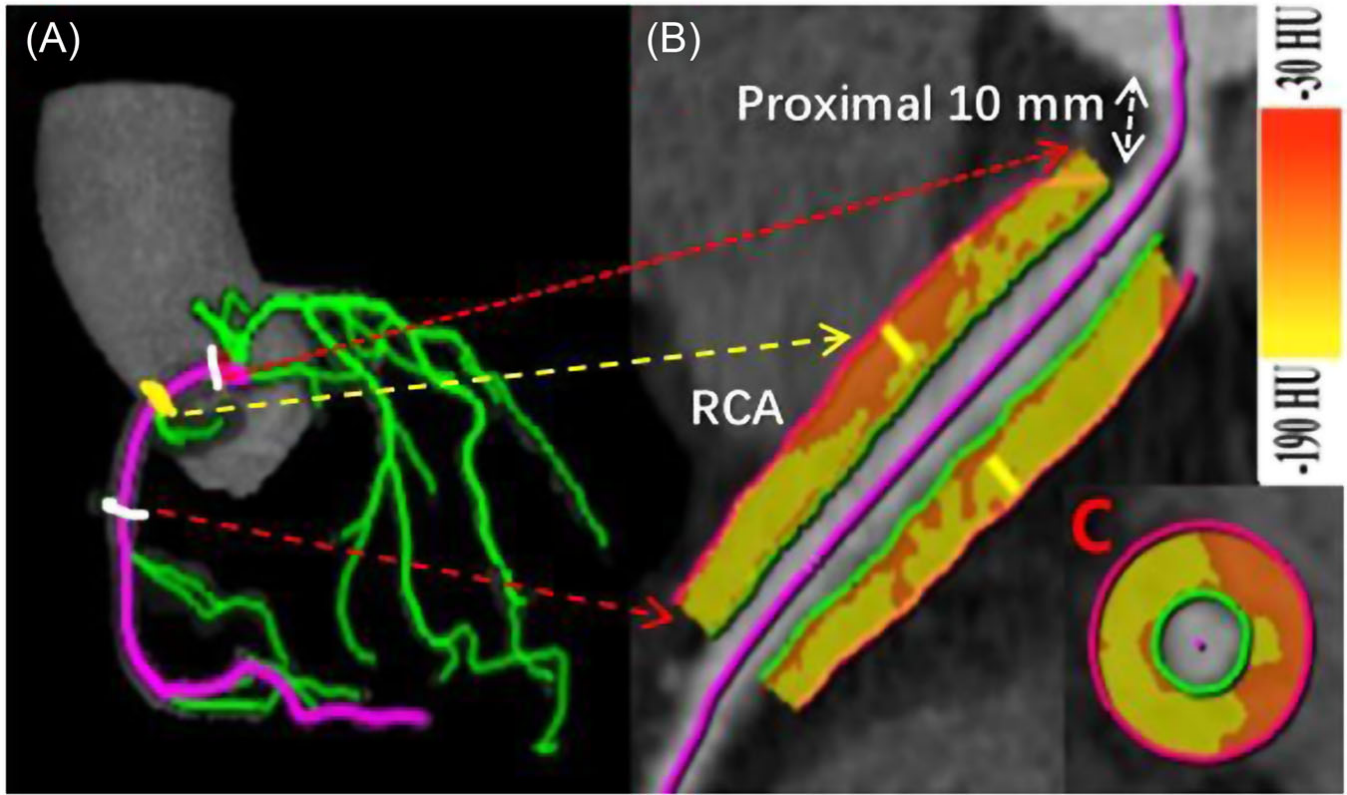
Semiautomated PCATA of RCA analysis on coronary computed tomography (CT) angiography. (A) Three‐dimensional reconstruction of all three major epicardial coronary vessels. (B) The PCAT phenotyping around the proximal RCA 10−50 mm segment was traced for the vessel. (C) The color map of coronary CT of PCATA in the cross‐sectional view. PCAT was defined as fat within a radial distance equal to the diameter of the vessel. AF, atrial fibrillation; HU, Hounsfield unit; PCAT, pericoronary adipose tissue; PCATA, pericoronary adipose tissue attenuation; RCA, right coronary artery.
